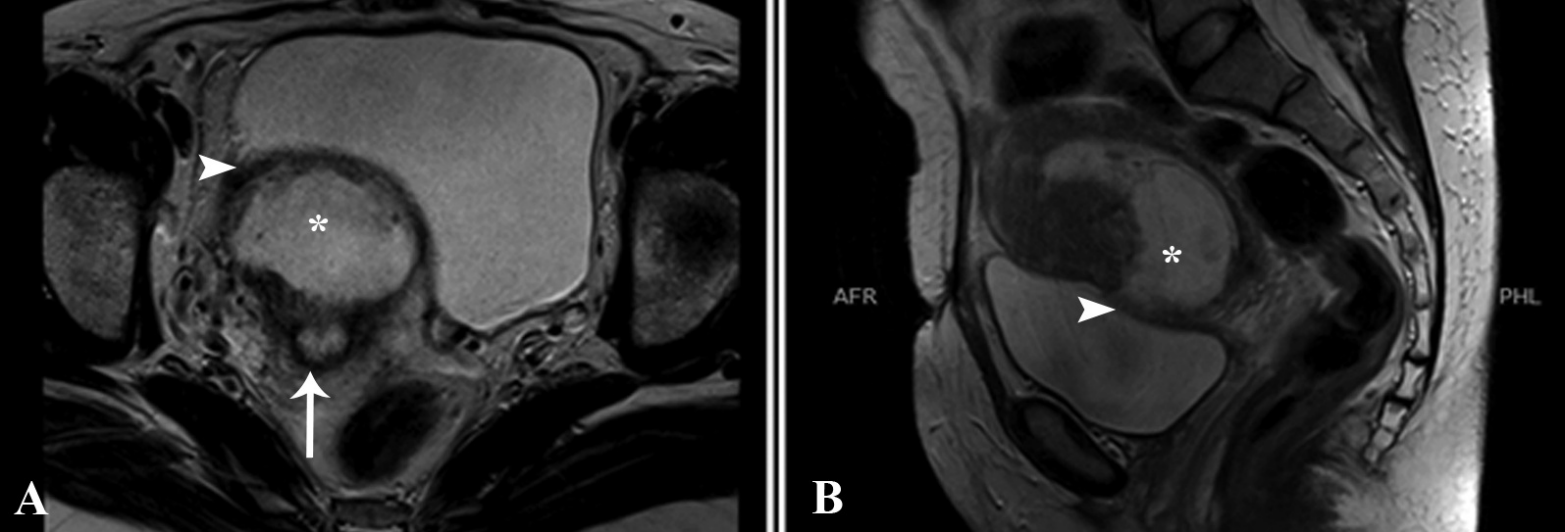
Axial and sagittal T2-weighted MRI from the pelvis showing gestational sac with fetal pole bulging anteriorly into the urinary bladder at the previous scar (asterisk). No myometrium identified adjacent to the sac where it was covered by thin hypointense layer of serosa (arrowhead). Endometrium is visualized separately (arrow). MRI: Magnetic resonance imaging
